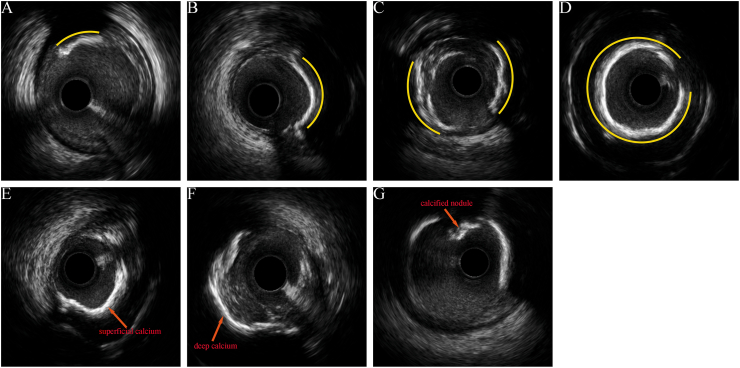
Types of coronary artery calcification on intravascular ultrasound. A, Grade 1 (0-90°); B, Grade 2 (91-180°); C, Grade 3 (181-270°); D, Grade 4 (271-360°); E, Superficial calcium; F, Deep calcium; G, Calcified nodule. The yellow line outlines the calcific arc. Figures from authors' organization. (For interpretation of the references to colour in this figure legend, the reader is referred to the Web version of this article.)
